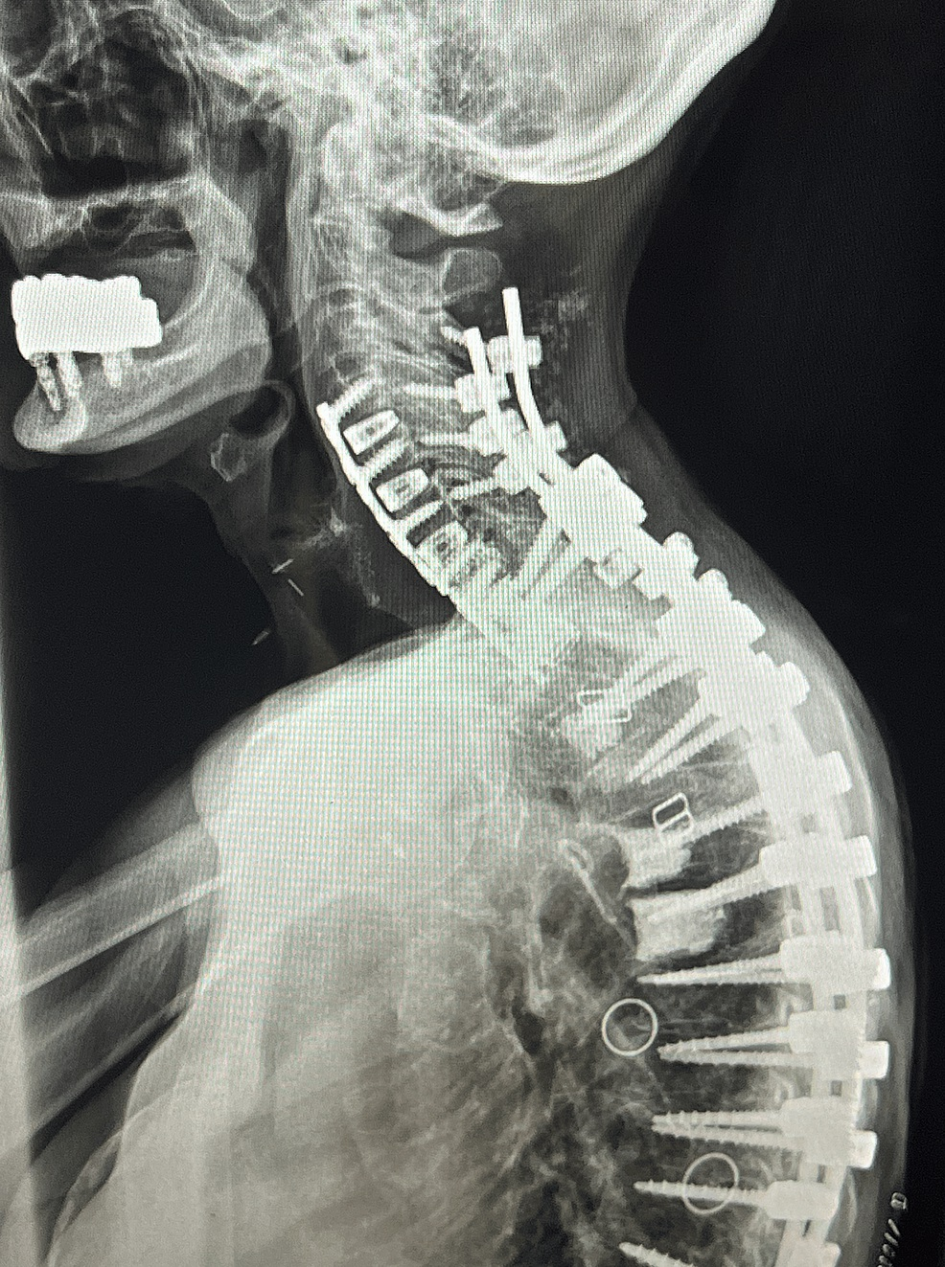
Lateral postoperative plain film imaging. This is a lateral radiograph of the cervical and thoracic spine demonstrating the postoperative double-plate technique.
